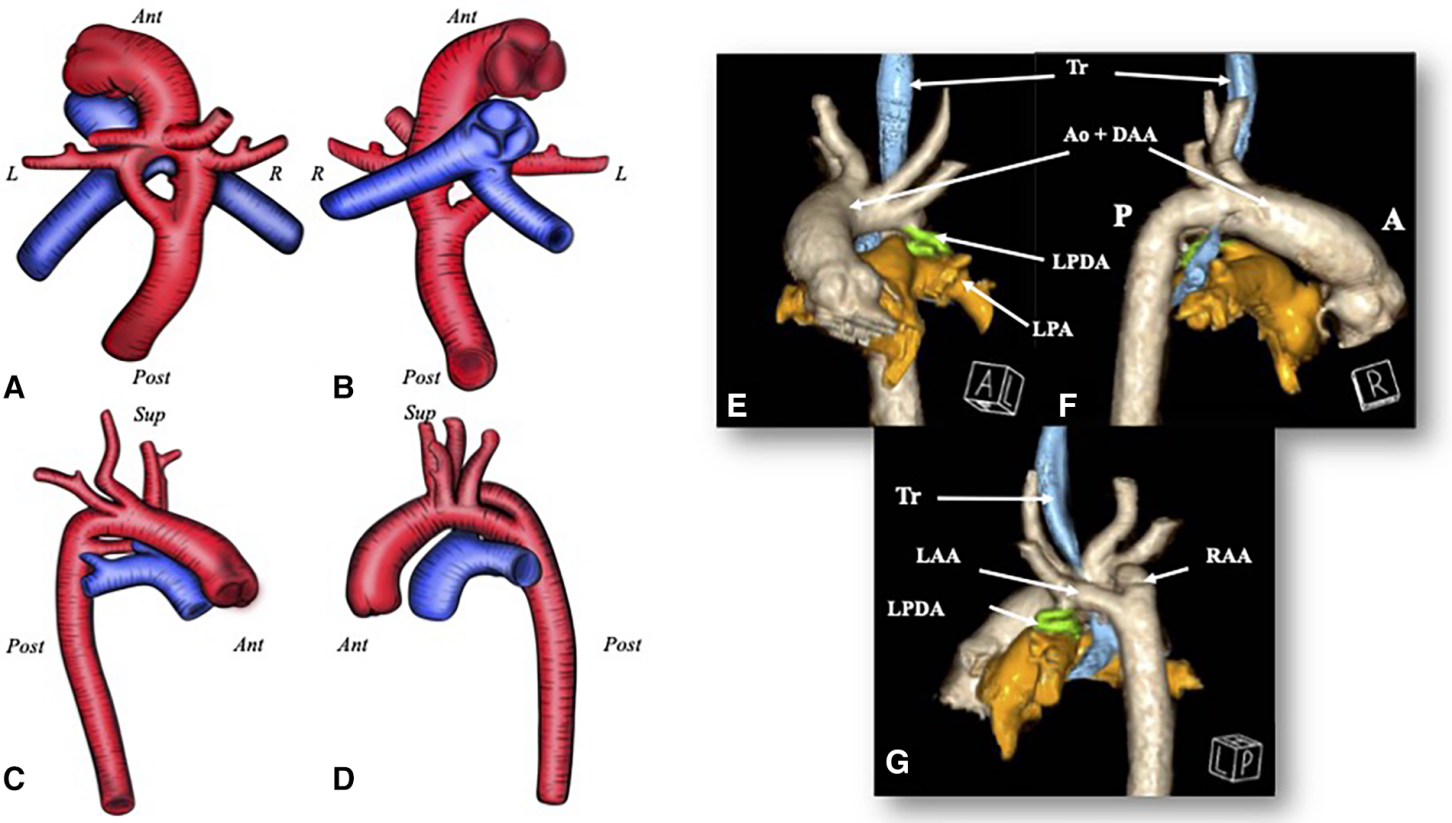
A D-TGA with a DAA. Image (A–D) The red blood vessel is the aorta with a double aortic arch; the blue one is the pulmonary artery. (A) A view from above; (B) a view from below; (C) a view from the right side; and (D) a view from the left side. (E–G) A CT scan 3D rendering of the double aortic arch in the transposition of the great arteries and the relationship with the trachea. (E) An anterior view; (F) a view from the right side; and (G) a posteroleft view. Ant, anterior; Post, posterior; R, right; L, left; Ao, aorta; LPA, left pulmonary artery; LPDA, left patent ductus arteriosus; Tr, trachea.
